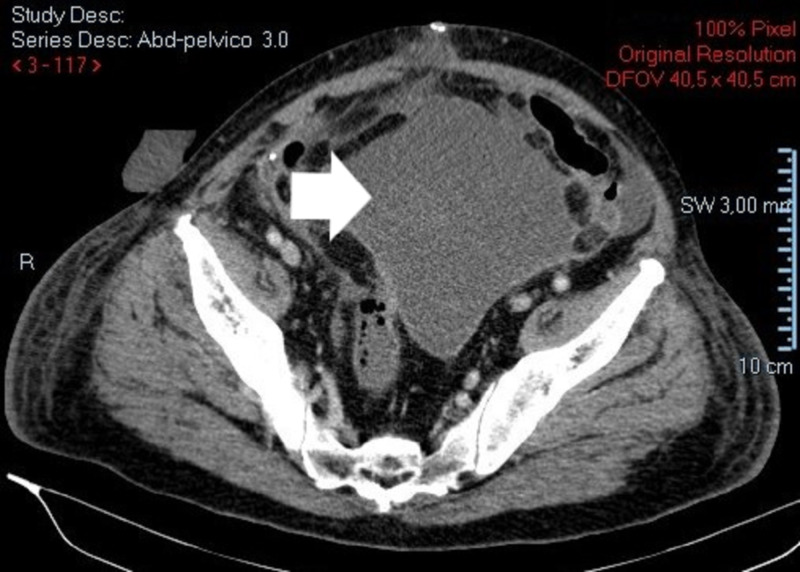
Contrast enhanced abdominopelvic CT scan. Intraperitoneal loculated free fluid.
